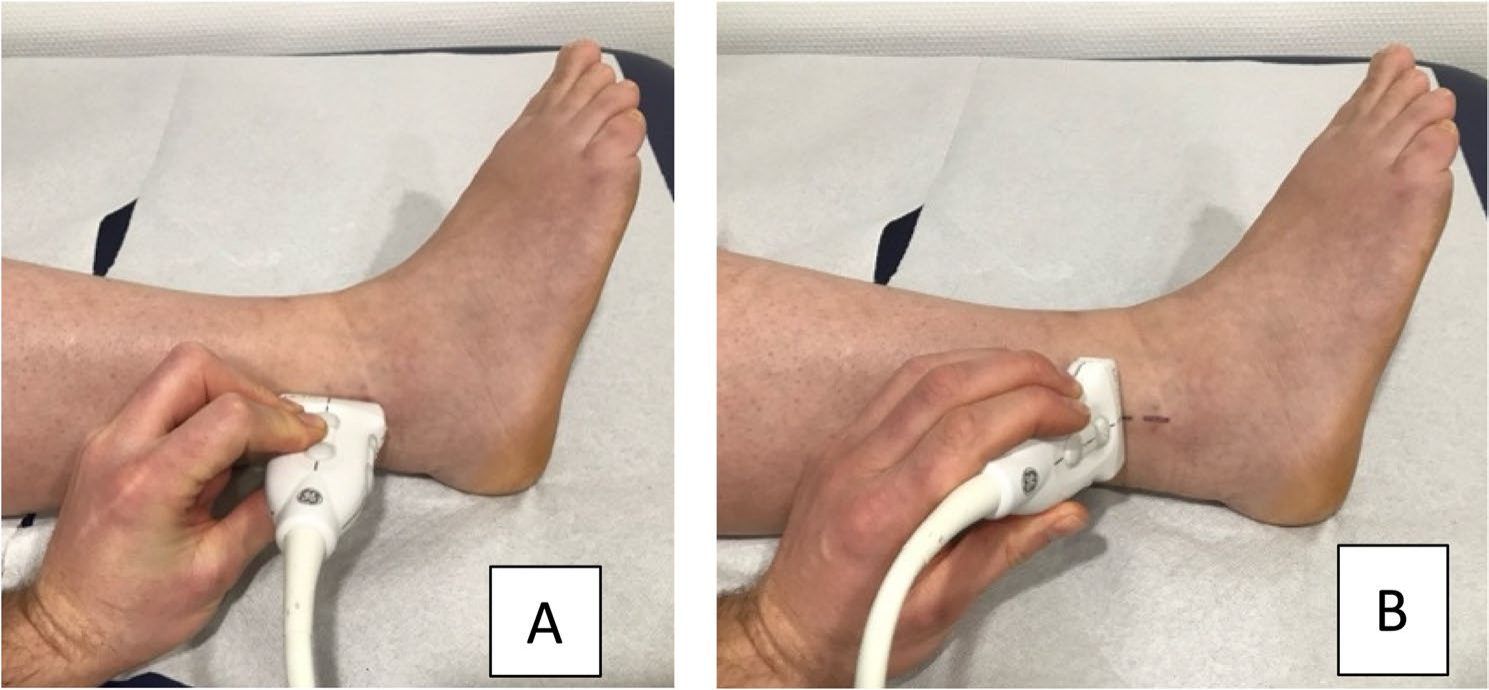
Preoperative ultrasound-guided localization of syndesmotic screws for surgical removal. The two clinical images demonstrate correct positioning of the ultrasound transducer over the lateral ankle: A in the longitudinal plane; B in the transverse plane for orthogonal confirmation and precise cross-referencing of the screw location. Skin marking is performed at the intersection of the two planes to guide incision. The images correspond to a 52-year-old female patient (BMI 33.2) with a history of ankle sprain and syndesmotic injury treated by syndesmotic screw fixation.
